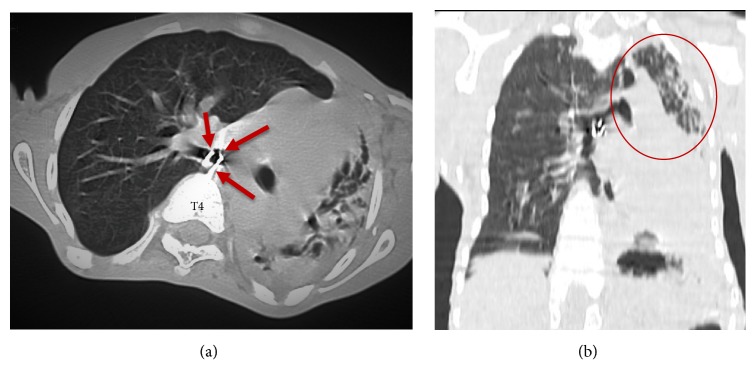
((a) = axial section and ((b) = coronal reconstruction):* Linear thick extraskeletal, parietal, tracheal, and left bronchial strain calcium formation, circumferential with intraluminal extension, attached to the anterior surface of the T4 vertebral body by a thick extension ((a): red arrows). CT in pulmonary window: atelectasis of the left pulmonary lobes ((b): red circle).
